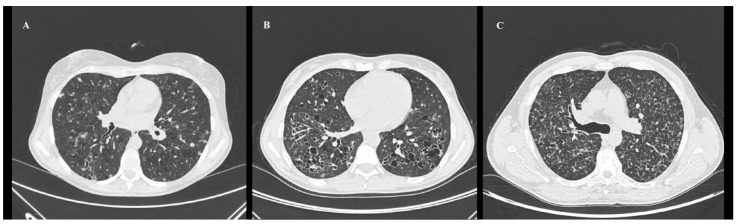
Chest HRCT, axial planes, shows PLCH in early, advanced, and end stage (A–C). (A): In early stage disease, there are nodular lesions corresponding to “florid” granulomas, characterized by brochiolocentric and ill-defined micronodules surrounded by ground-glass opacification secondary to inflammatory interstitial infiltration, nodules with irregular margins and centrilobular distribution, and cavitary nodules with thick walls that later become cysts. (B): In advanced stage disease, cystic lesions usually predominate on nodules: cysts appear round and of small dimension (<10 mm), but in advanced disease they are typically larger and of different shapes with thick-walled cysts (>2 mm thick) that progressively transform into thin-walled cysts (<2 mm thick). (C): In end-stage disease, there is a fibrocystic pattern that maintains the typical upper- and middle-lung zone predominance.
